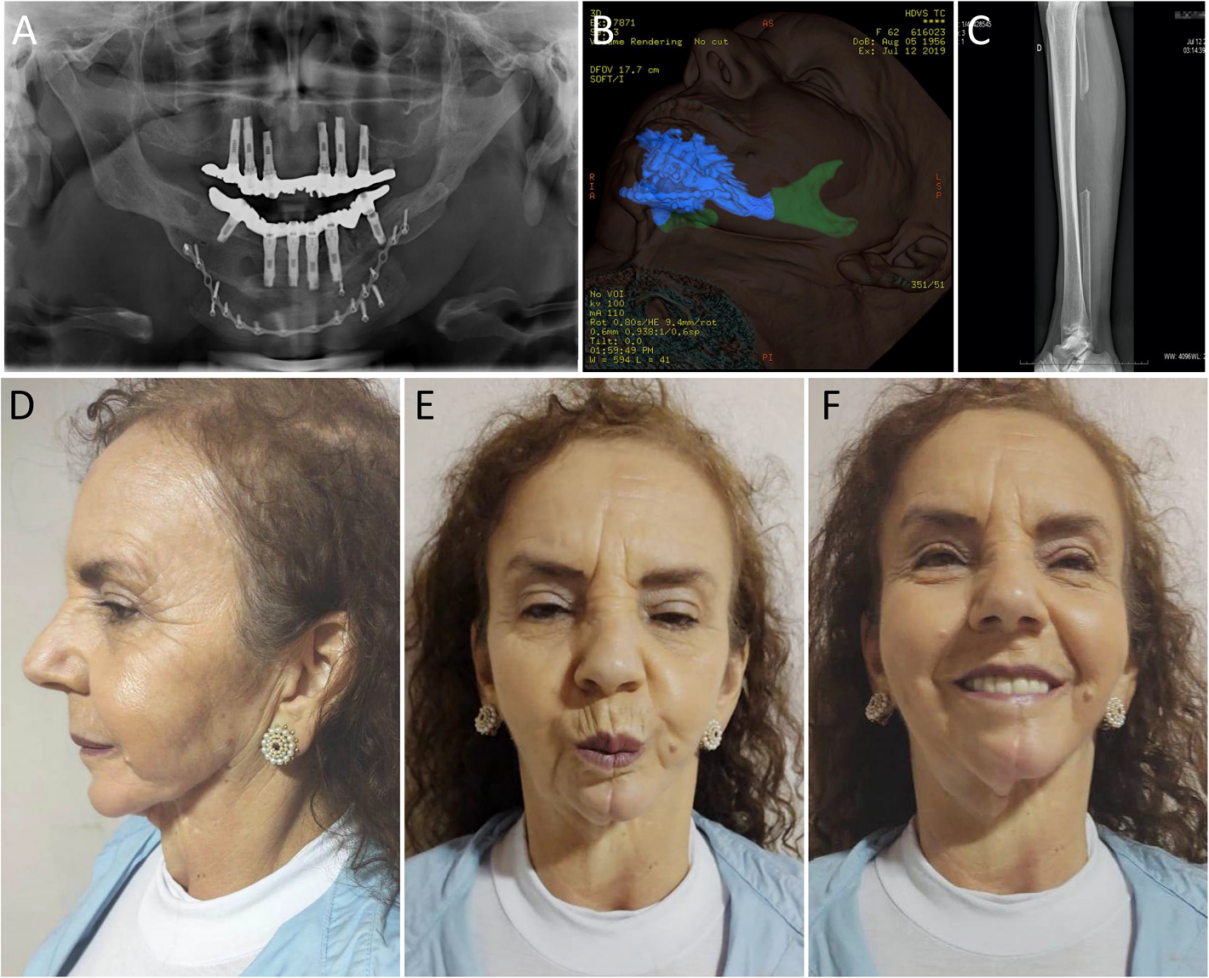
Postoperative aspects, in long-term follow-up of mandibular reconstruction with FVFF. a Panoramic radiograph showing implant-supported protocol-type prosthesis and a 16-hole 2.0 long plate used for fixation of the rib graft. b Facial 3D computed tomography (CT), showing the integration of the fibula, iliac crest and rib grafts (in blue) to the mandible remnant (in green). c Radiograph of the donor area, right leg. d, e, f Facial aspects. Images from 2021
